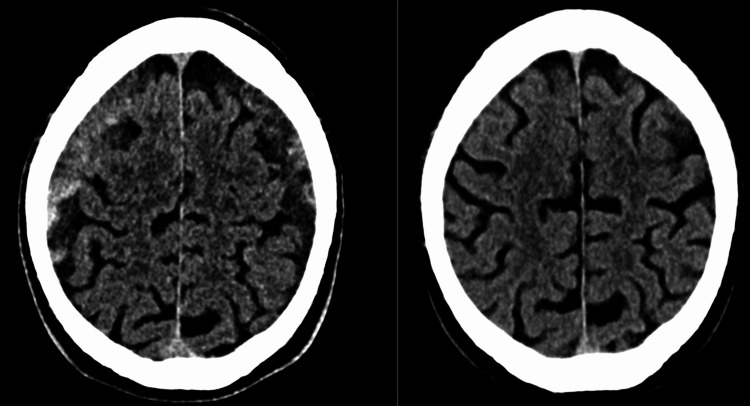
Pre- (left) and post-intervention (right) CT scan images of a patient who underwent craniotomy, as well as a middle meningeal artery (MMA) embolization. The right post-intervention image at three months indicates a clear resolution of the bilateral subdural hematoma (SDH).
